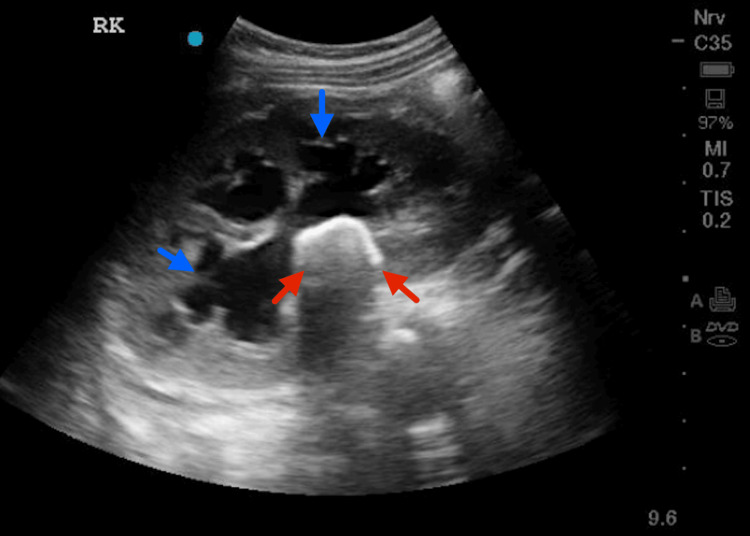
Ultrasound of the right kidney demonstrating renal calculi with hydronephrosis The blue arrows point to the dilated renal calyxes, and the red arrows point toward the renal calculi with distal hypoechoic shadows.
